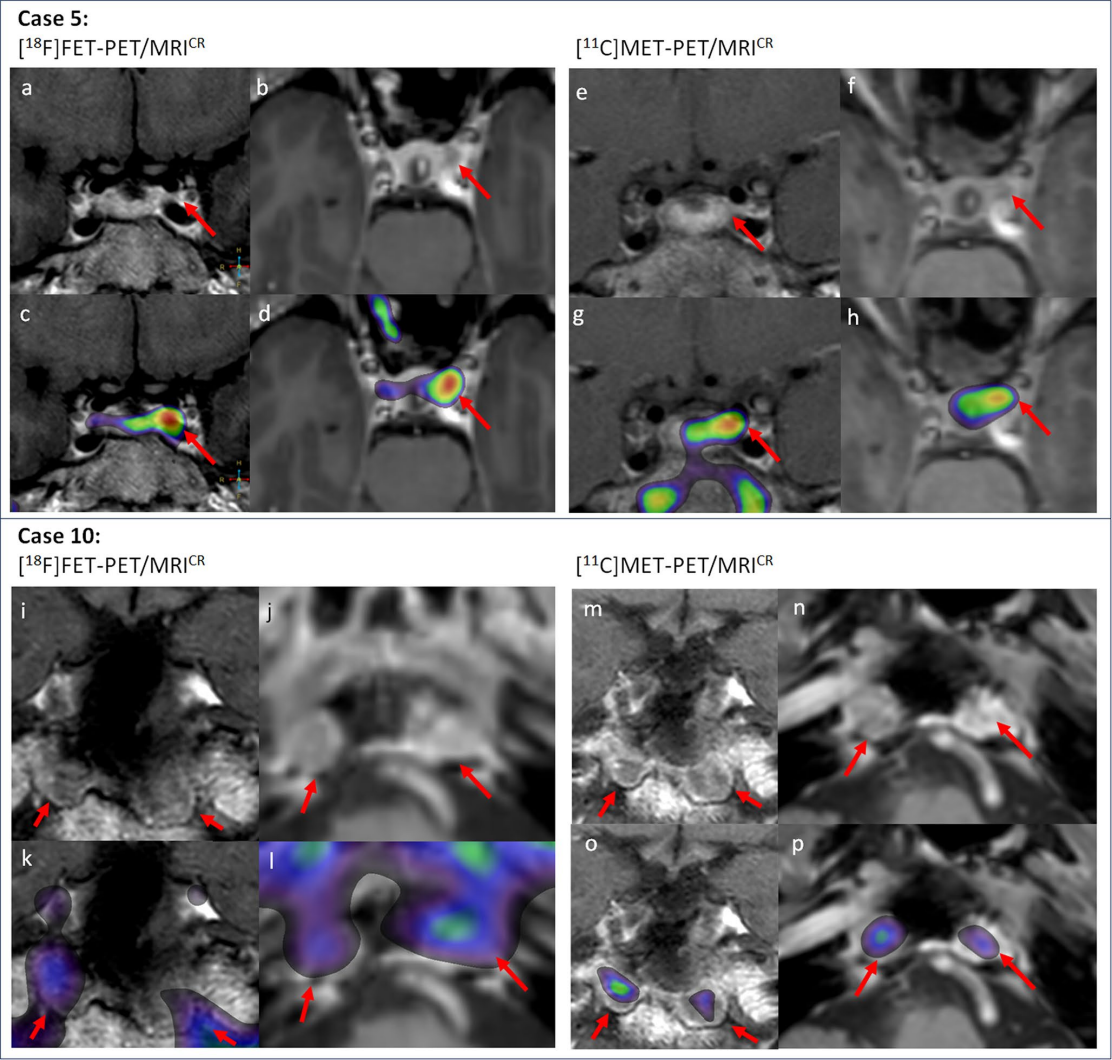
[^18^F] FET-PET/MRI^CR^ and [^11^C]MET-PET/MRI^CR^ images. Case 5: Female patient who underwent [^11^C]MET-PET/MRI^CR^ due to persistent disease post-surgery (2019). (e) Coronal T_1w_ MRI (post-contrast) with the suspected residual adenoma left anterolateral in the sella with extension in the cavernous sinus (f) axial T_1w_ MRI (post-contrast) showed the suspected lesion left anterolateral in the sella, (g) [^11^C]MET-PET/MRI^CR^ fusion (coronal) showed increased activity in the suspected lesion, (h) [^11^C]MET-PET/MRI^CR^ fusion (axial). Following this imaging biochemical surveillance was continued due to the mildness of symptoms and a low likelihood of total resection. In 2021 new irregularity of the patient’s menstrual cycle necessitated reevaluation of treatment options. Sequential MRIs raised the suspicion of multifocality, for which [^18^F]FET-PET/MRI^CR^ was performed (a) Coronal T_1w_ MRI (post-contrast) with suspected residual adenoma left anterolateral in the sella with extension in the cavernous sinus, (b) axial T_1w_ MRI (post-contrast) showed the suspected lesion left anterolateral in the sella, (c) [^18^F]FET-PET/MRI^CR^ fusion (coronal) confirms the location of the suspected lesion with increased focal activity left anterolateral in the sella, (d) [^18^F]FET-PET/MRI^CR^ fusion (axial). *Case 10*: Male patient with residual disease after two surgeries. [^18^F]FET-PET/MRI^CR^ was performed to identify the most active location of residual tissue for future treatment (TSS or radiotherapy). (i) Coronal T_1w_ MRI (post-contrast) with two possible locations in the sella floor with extension into the destructed clivus, (j) axial T_1w_ MRI (post-contrast), (k) [^18^F]FET-PET/MRI^CR^ fusion (coronal) showed diffuse moderate activity on both sides in the sella floor, possible locations of residual adenoma, yet inconclusive due to diffuse tracer uptake (10 weeks postoperative), (l) [^18^F]FET-PET/MRI^CR^ fusion (axial). Nine months later [^11^C]MET-PET/MRI^CR^ was performed for confirmation. (m) Coronal T_1w_ MRI (post-contrast) showed the two possible locations adjacent to the sphenoid sinus with extension into the destructed clivus, (n) axial T_1w_ MRI (post-contrast), (o) [^11^C]MET-PET/MRI^CR^ fusion (coronal) with clearly increased focal uptake in the suspected locations, on the right more than on the left side (p) [^11^C]MET-PET/MRI^CR^ fusion (axial).
